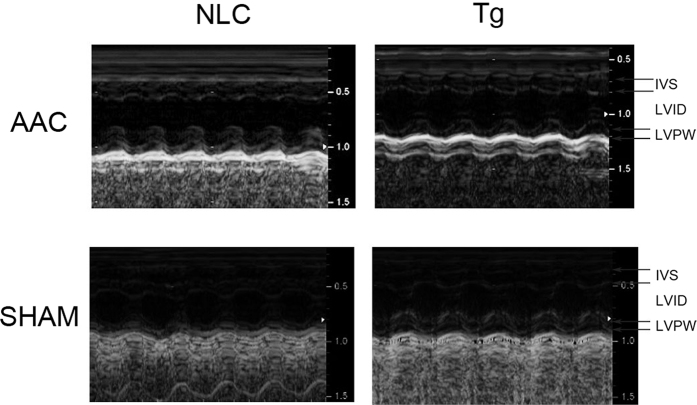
M-mode pictures from the echocardiography. NLC, non-transgenic littermate control; Tg, transgenic; AAC, abdominal aortic constriction. IVS, interventricular septum thickness; LVPW, left ventricular posterior wall thickness; LVID, left ventricular internal dimension.
